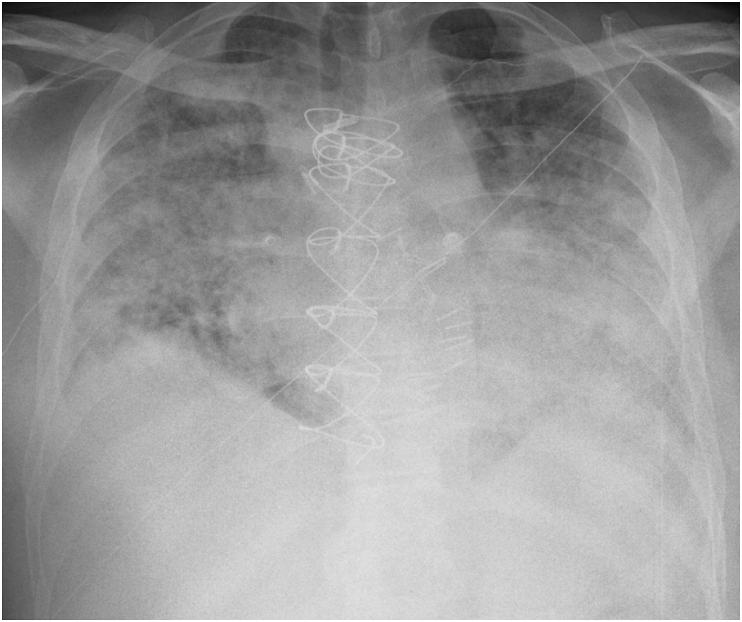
Chest radiograph of Case 1 on day of admission which shows bilateral multifocal airspace opacities.
